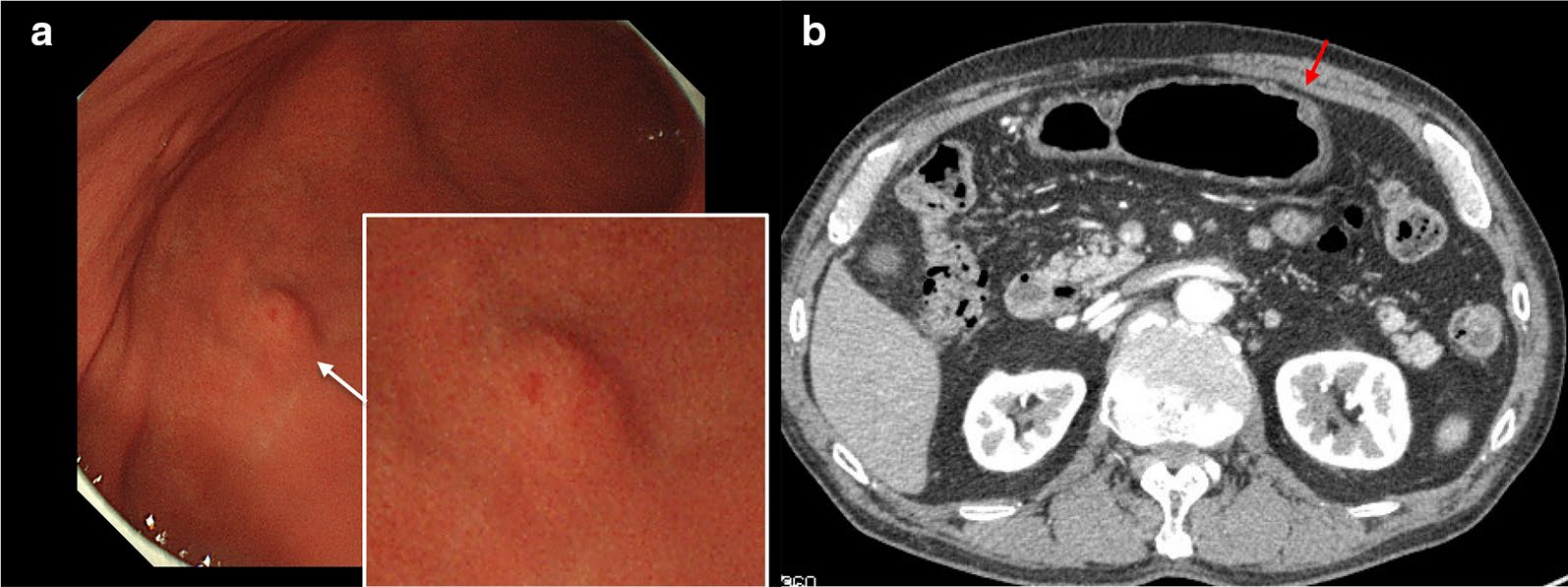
a An SMT 7 mm in diameter was revealed at the greater curvature of the angulus by upper gastrointestinal endoscopy. b The SMT was detected by contrast-enhanced CT
